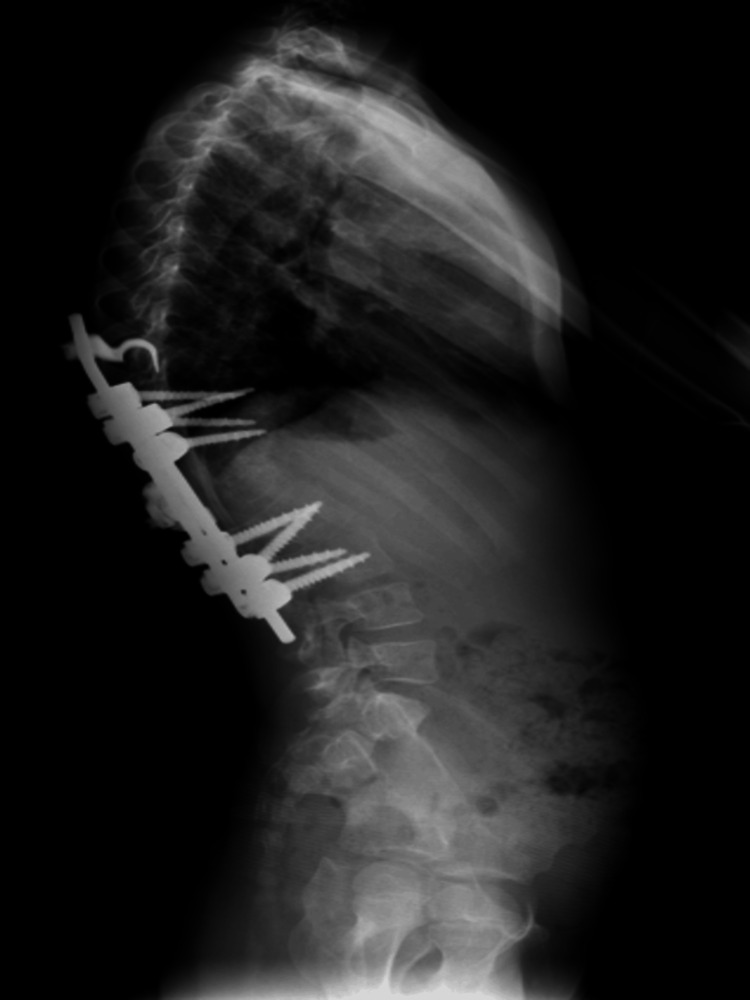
A post-operative lateral radiograph of the thoracolumbar spine depicts the T10-L2 spinal fusion with pedicle screws, the T9 hook, and the resection of T12.
